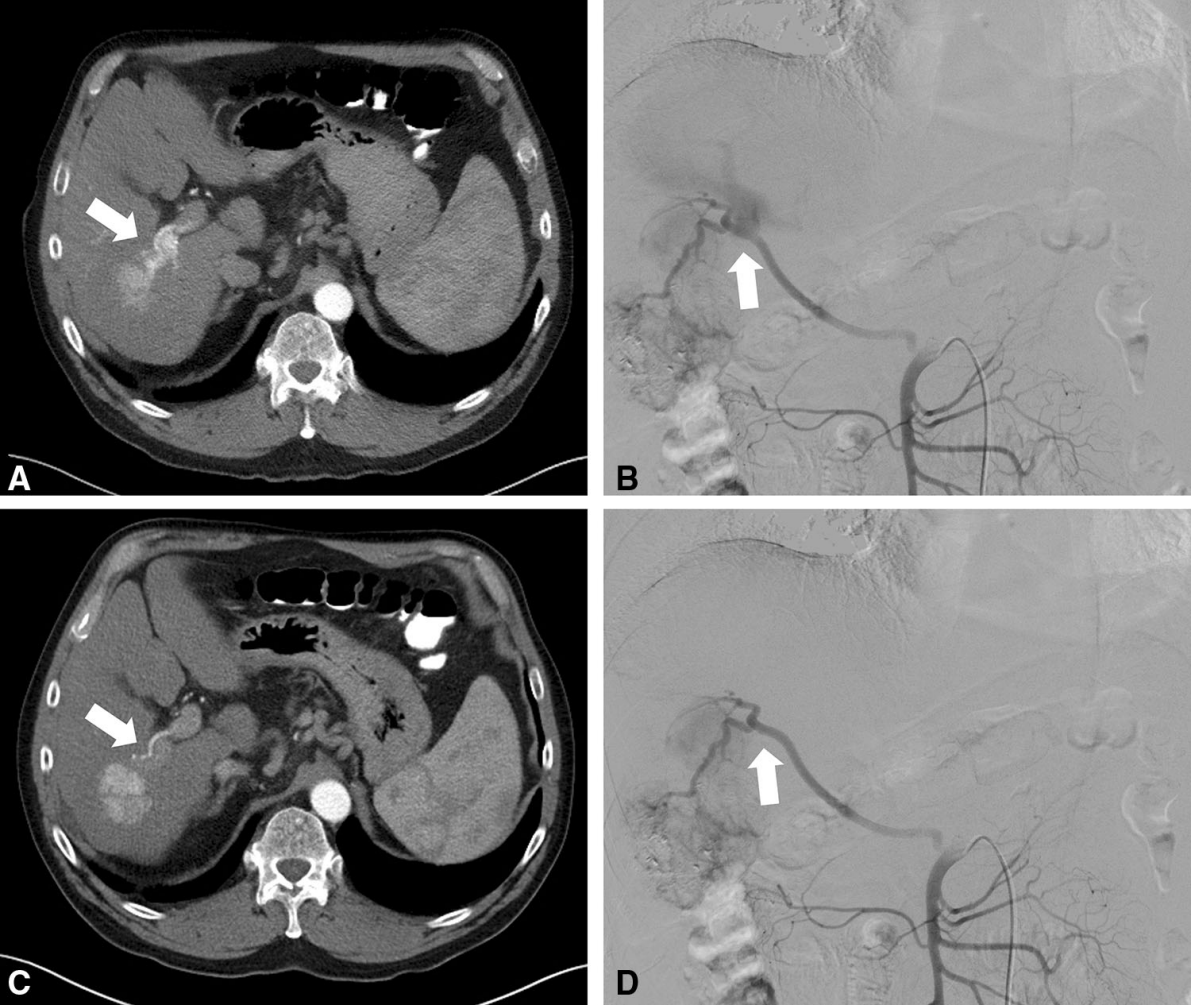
A 65-year-old patient with multifocal HCC based on ethyl toxic liver cirrhosis. A An arterioportal fistula (arrows) can be delineated based on the CT image. B DSA confirms the large arterioportal fistula (white arrow) with high-flow conditions. C After the treatment with sorafenib, the fistula cannot be visualized (white arrow). D Technical, uncomplicated and safe TACE could be carried out under occluded fistula (white arrow)
